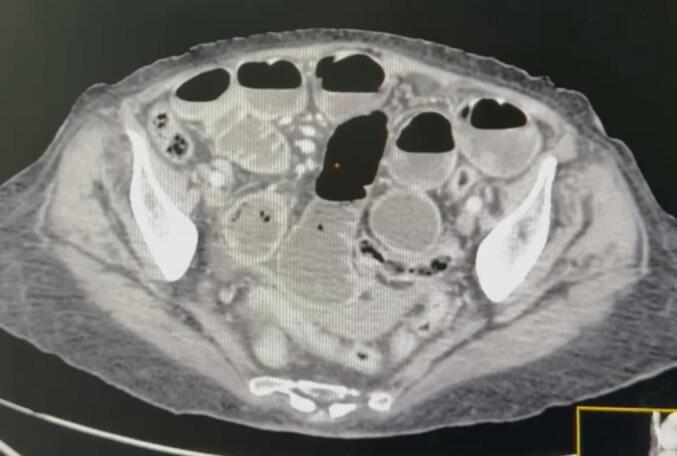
Axial CT scan demonstrating significant small bowel distension.
